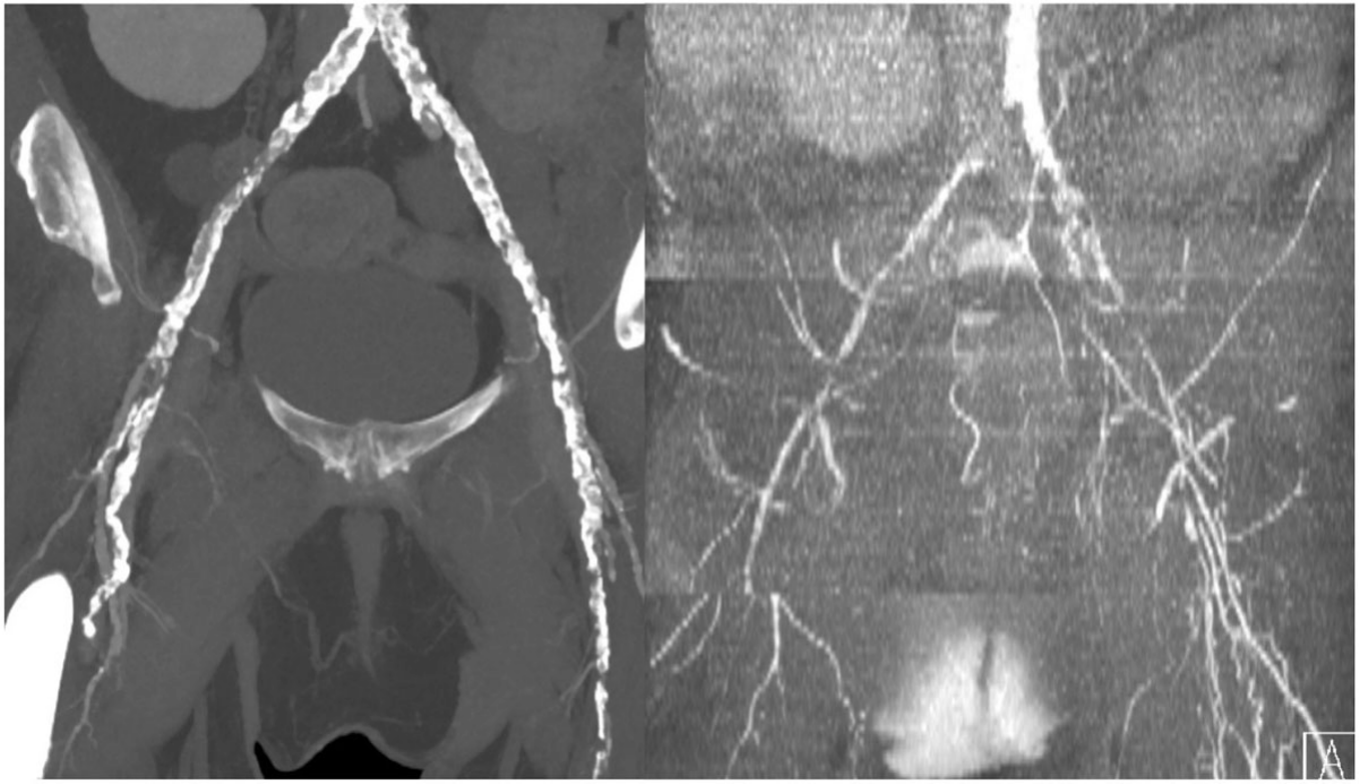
MIP CTA and MIP QISS MRA in a 54 yo female. CTA demonstrates the arterial system and calcifications, but extensive atherosclerosis limits evaluation of the vessel lumen. Absent flow in the proximal right common iliac artery is well identified by QISS MRA.
